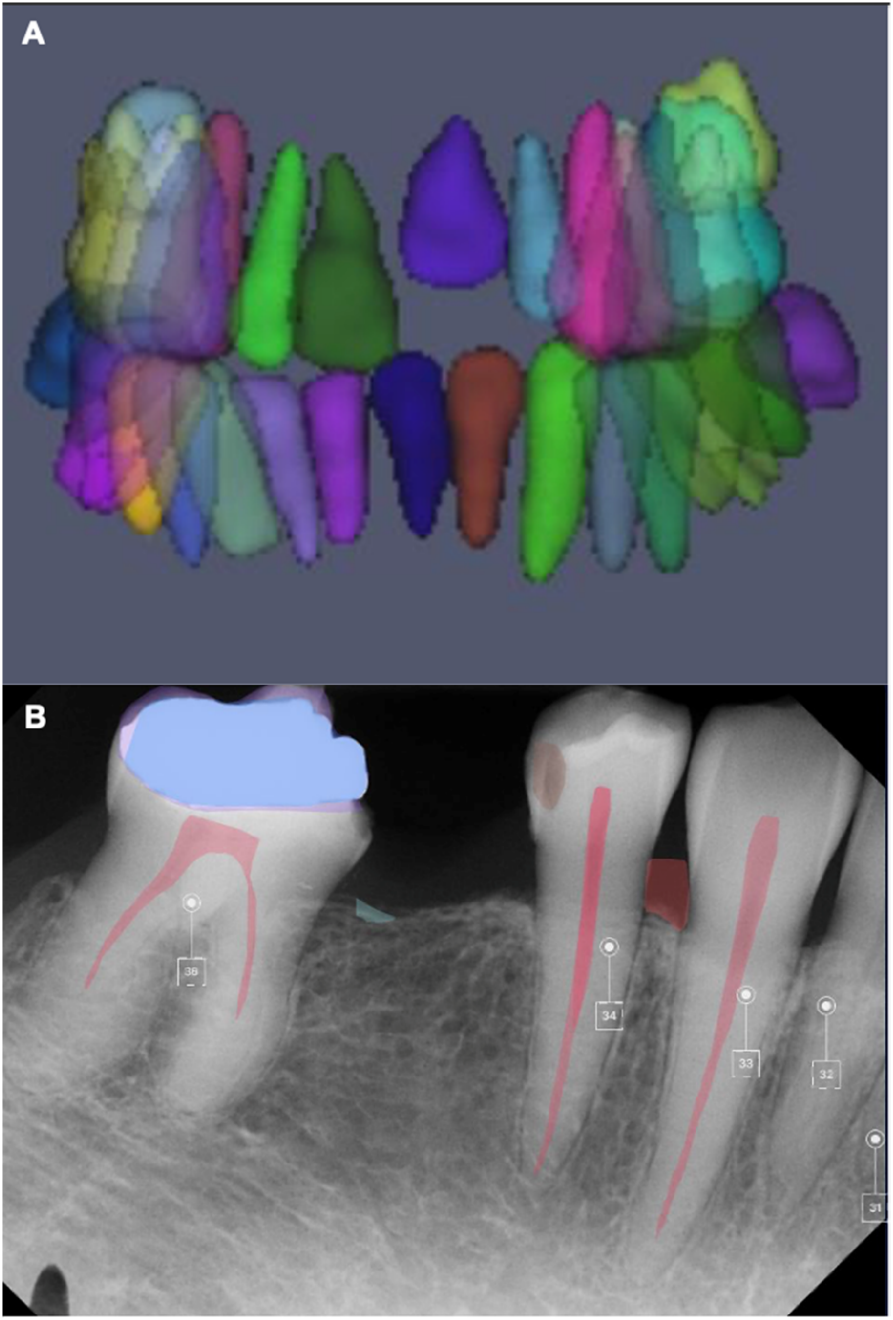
AI for tooth presentation in 3D view by an AI system (A) and automatic crown and caries detection on the periapical radiograph (B) (courtesy—cranioCatch AI software).
